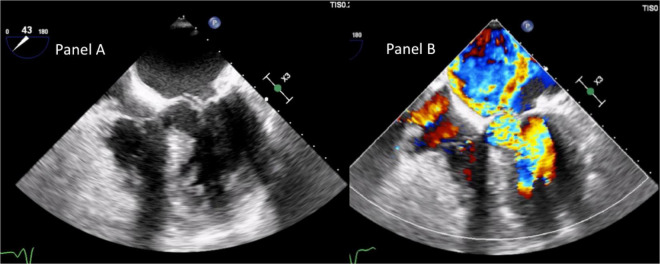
Trans-esophageal echocardiogram mid-esophageal 5-C view demonstrating, in panel (A), basal interventricular septum severe hypertrophy, SAM of AML, chordal rupture, and the related PML-flail. Panel (B) shows the two different jets there were at color Doppler. The posteriorly directed jet was due to SAM-related MR. The anteriorly directed jet was related to PML-flail.
